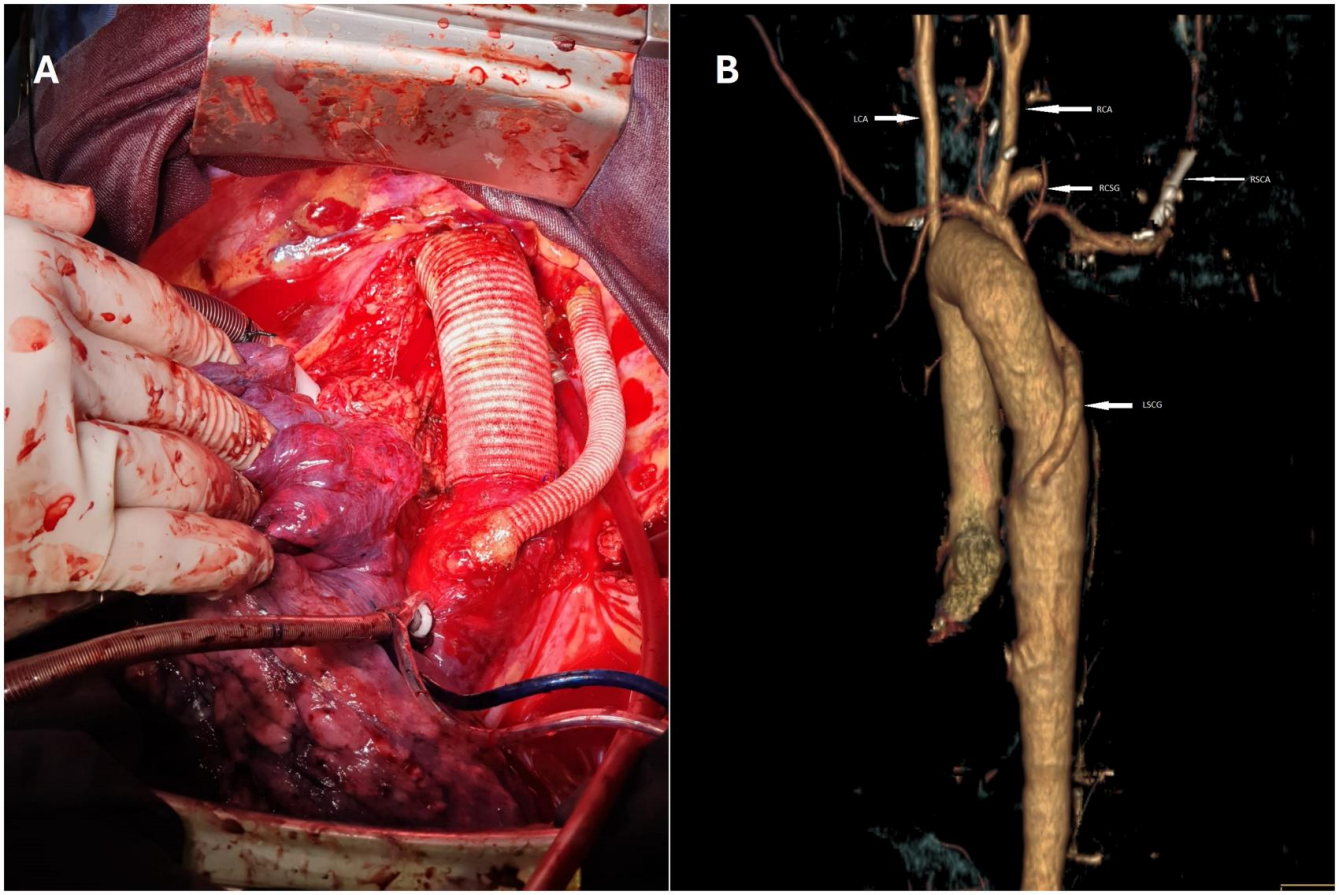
(A) Intraoperative view of the final result. (B) 3D reconstruction of CT angiography 1-year post-surgery. LCA: left carotid artery; LSCG: left subclavian artery graft; RCA: right carotid artery; RSCA: right subclavian artery; RCSG: right carotid subclavian graft.
